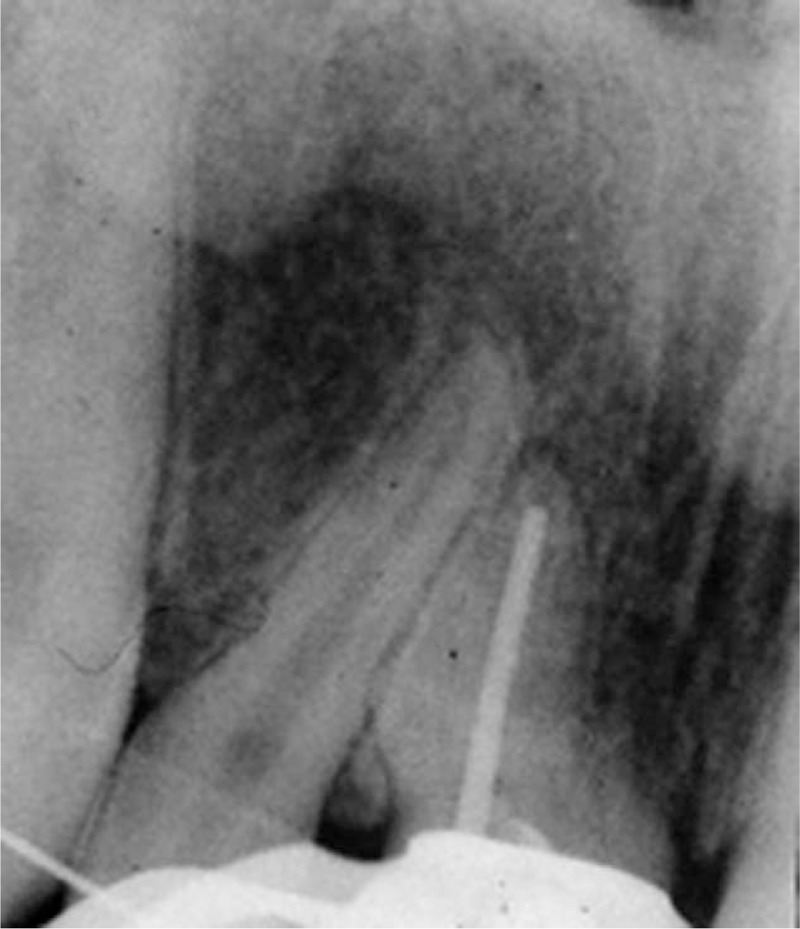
Radiographic control of the apical position of the master cone. It confirmed that the preparation was correct, reaching 1 mm from the root apex.
